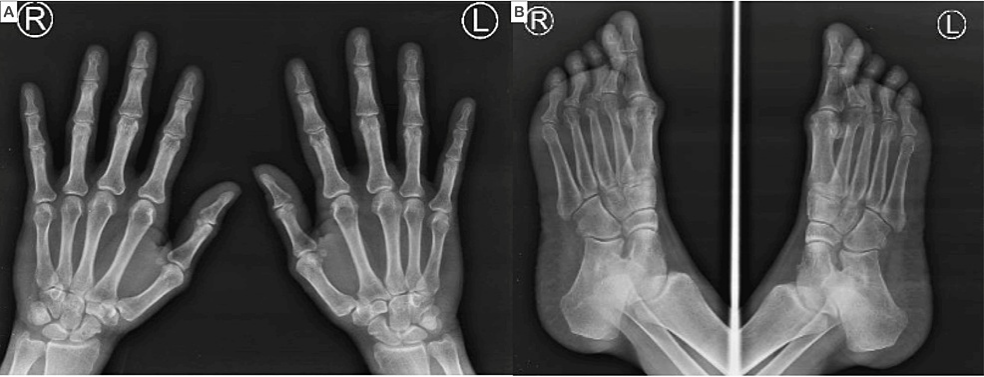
X-rays of hands and feet Normal hands and feet X-rays, without signs suggestive of rheumatoid arthritis.
